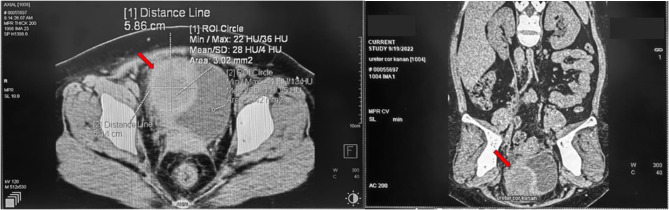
CT scan of the abdomen with contras demonstrating a bladder wall abscess ((a) red arrow) and a 5.86 × 5.14 cm delta 28 HU density in the right anteroinferior bladder wall
